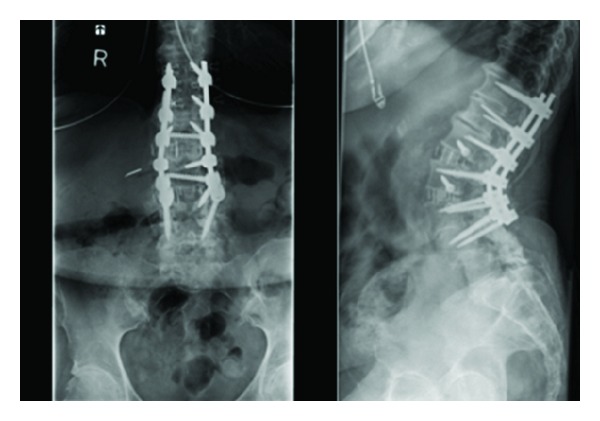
AP and lateral radiographs of the lumbosacral spine showing interbody fusion at L1-L2, and L2-L3 via the lateral approach and extension of posterior instrumentation and fusion from T11-L4. Notice improvement of lumbar lordosis and height restoration at the neuroforamina of L1–L3.
